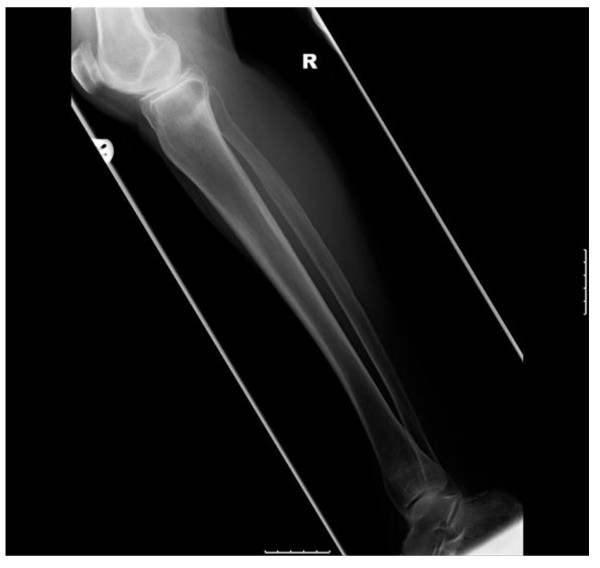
Lateral radiograph of the right tibia six months after diagnosis with resolution of symptoms and healed fractures.
